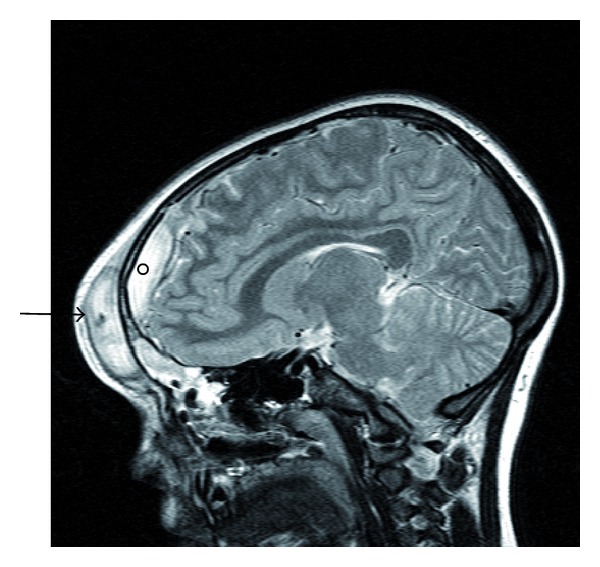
Sagittal MRI demonstrating an abscess within the frontal scalp (arrow) and an adjacent epidural abscess (circle).
